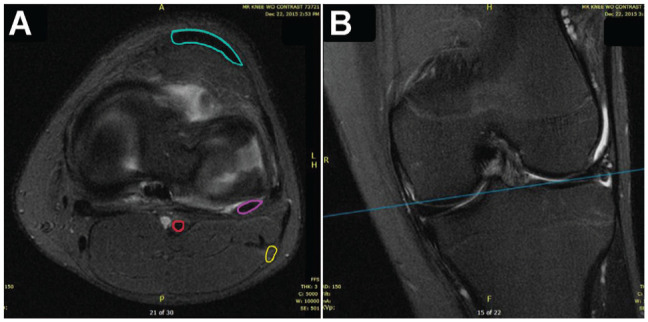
(A) Axial and (B) coronal side-by-side MRI sequences at the level of the lateral meniscus. Line in (B) is the correlated level of the axial image in A. Outlined in (A) are the patellar tendon (blue), popliteal tendon (purple), common peroneal nerve (yellow), and popliteal artery (red).
